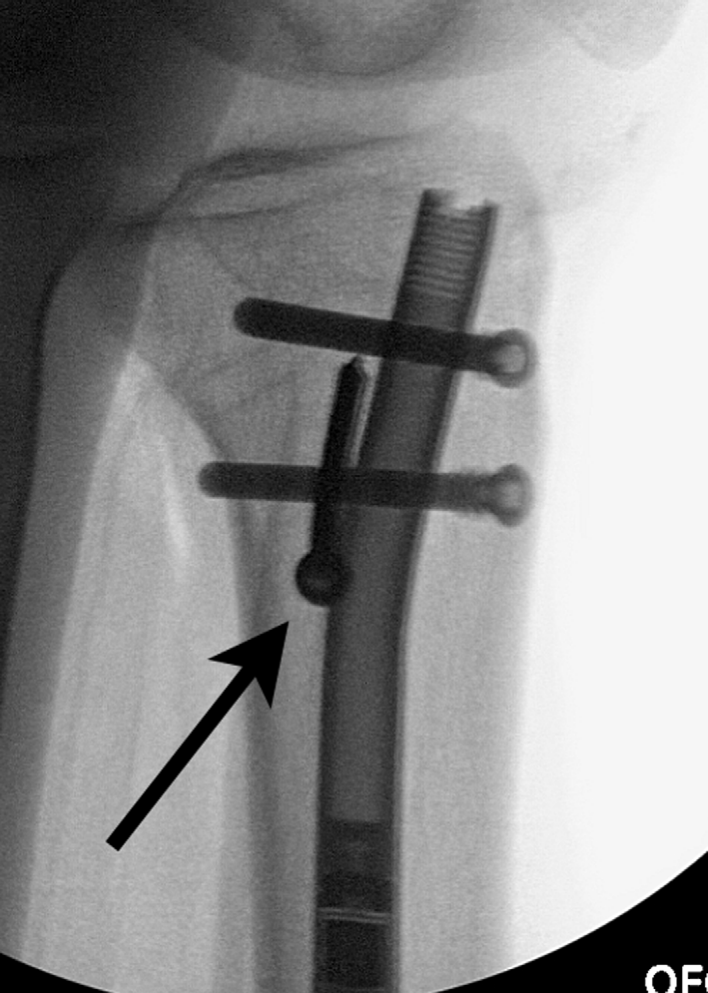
In this unrelated case, the proximal syndesmotic screw also served as the posterior blocking screw (arrow).Fasciotomy: It is routine to perform a percutaneous fasciotomy of the anterior compartment when acutely correcting a tibial deformity to prevent compartment syndrome [18].Follow-up: Lengthening begins after a latency period and proceeds at approximately 0.75 mm per day. The patient is followed at regular intervals with radiographs checking for regenerate quality and joint contractures (Figures 15a and 15b).Figure 15. F15:
